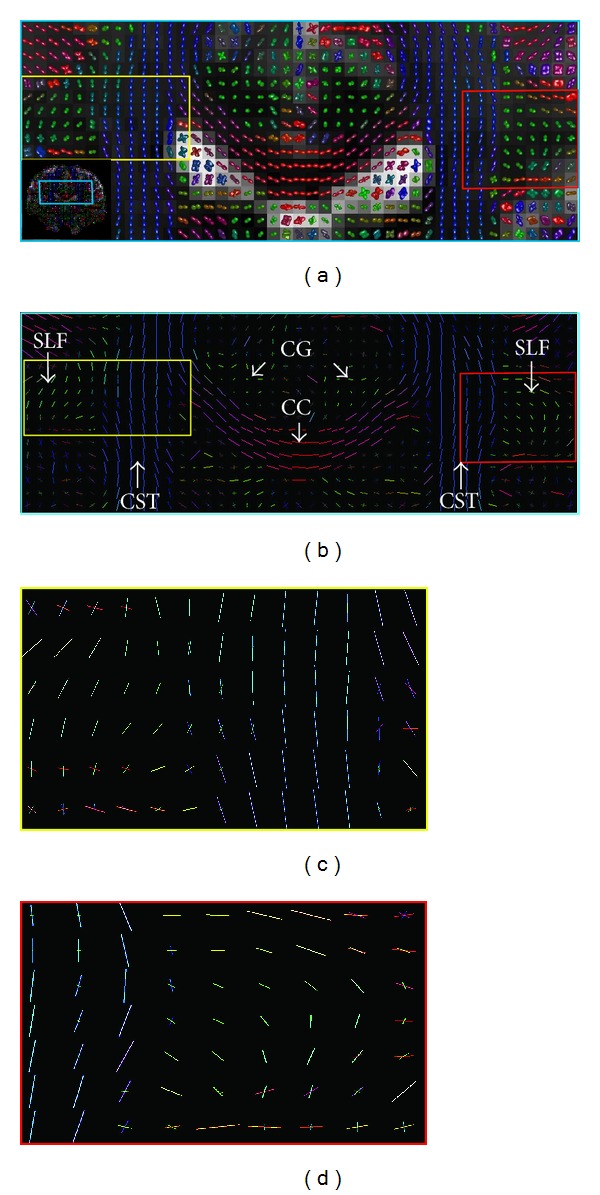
(a) Fourth order CT-FODs are reconstructed from* in vivo* human cerebral dataset and represent the Corpus Collum (CC), the Corticospinal Tract (CST), the Cingulum (CG), and the Superior Longitudinal Fasciculus (SLF) on a coronal slice; the CT-FODs are superposed on the raw diffusion signal. (b) represents the fiber directions (colored according to their directions) extracted using the Adecomp-SHOT based approach. Yellow and red highlighted areas are, respectively, zoomed in (c) and (d).
